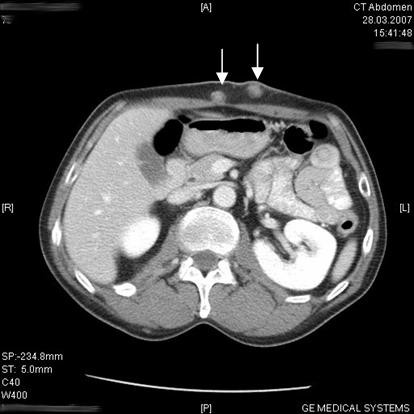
Thoraco-abdominal CT scan showing two representative cutaneous metastases (arrows).
